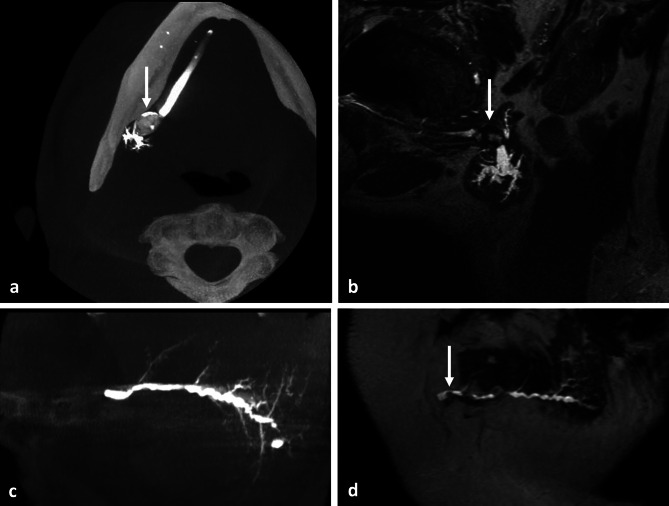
Identification of sialolithiasis. Large salivary stone (white arrow) located in the proximal third of the right Wharton’s duct, visible as calcified concretion within an opacified salivary duct with 3D-CBCT sialography in maximal intensity projection (MIP) axial view (a), and as a strong hyposignal with upstream hyperintense ductal dilatation in MR sialography in MIP sagittal oblique view (b). Case of discordance between the two imaging examinations with a distal sialolithiasis of the left Stensen’s duct undetected on the sagittal MIP view in 3D-CBCT sialography (c) and diagnosed on the sagittal MR sialogram (d)
